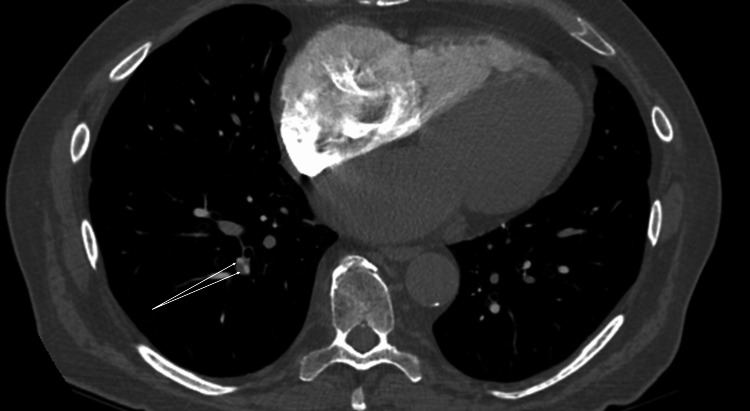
Chest CT PE protocol Chest CT shows a small filling defect on the right lower lung consistent with pulmonary arterial embolism (arrows). CT: computed tomography, PE: pulmonary embolism
